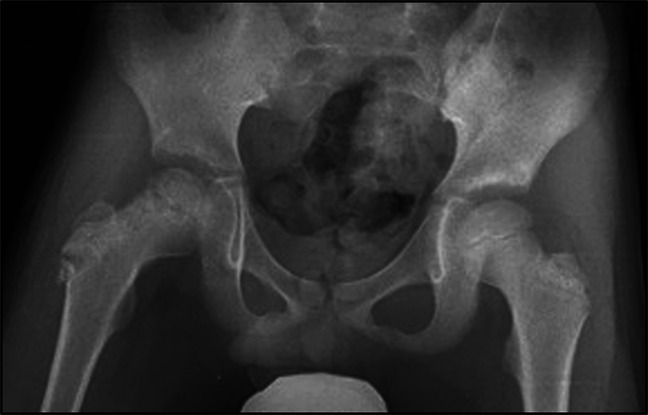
AP radiograph of the pelvis obtained 2 weeks after surgery showing postoperative changes and bone graft material filling the lesion within the epiphysis and the surgical tract within the femoral neck.
